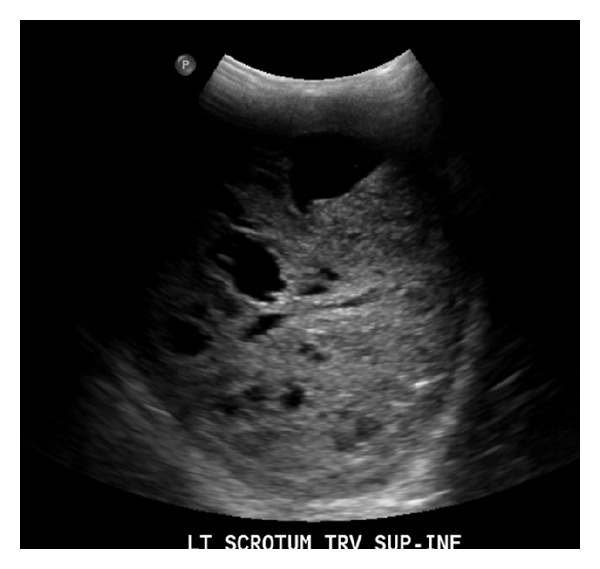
Ultrasound of left testis showing hypoechoic enlargement and scattered lobulated anechoic cystic foci of various sizes.
